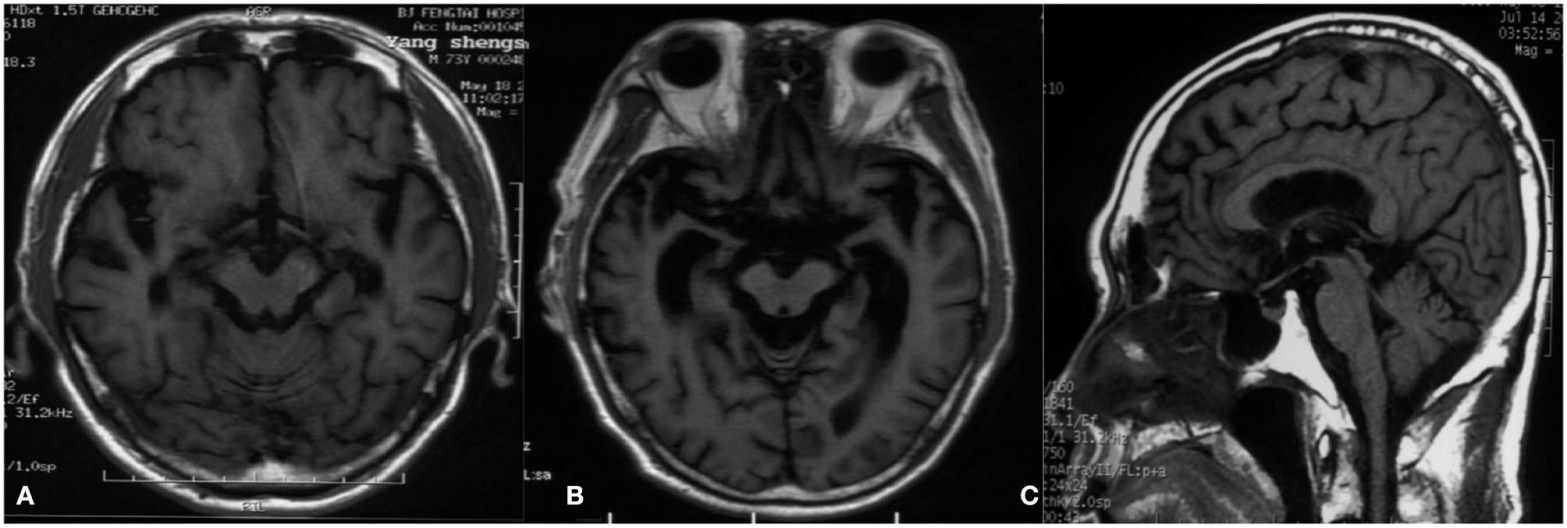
Magnetic resonance imaging. (A) T1-weighted image 2 months after the onset of the disease shows extensive cortical atrophy. (B) T1-weighted image 12 months after the onset of the disease reveals severe cortical atrophy, and the degree of atrophy gradually increased compared to A. (C) T1-weighted image 4 months after the onset of the disease demonstrates that the midbrain volume was comparatively well preserved compared with cortical atrophy.
